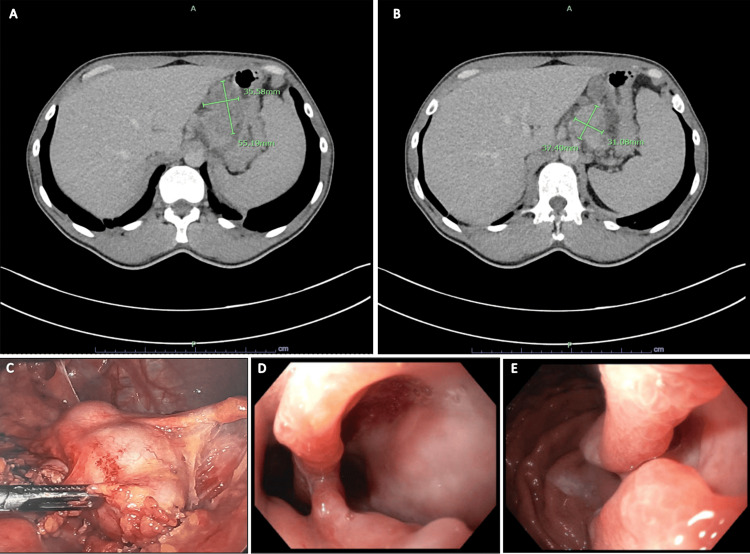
Initial evaluation with CT scan of the abdomen with IV contrast and endoscopic findings. (A) Soft tissue is seen along the gastro-hepatic ligament, which effaces along the subserosa surface of the lesser curvature of the stomach in the gastric body with a conglomerate measurement of approximately 3.6 × 5.5 cm. (B) Bulky gastro-hepatic lymphadenopathy measuring up to 3.1 × 3.7 cm. (C) Exploratory laparoscopy revealed bulky lymphadenopathy in the celiac trunk along the lesser curvature of the stomach compressing the left gastric artery. (D and E) Endoscopic findings revealed a fungating and ulcerating mass with bleeding upon contact at the GEJ, extending 40-45 cm from the incisors. The Z-line is located at 41 cm. The mass was non-obstructive and partially circumferential, involving one-third of the lumen circumference. CT: computed tomography, GEJ: gastroesophageal junction
